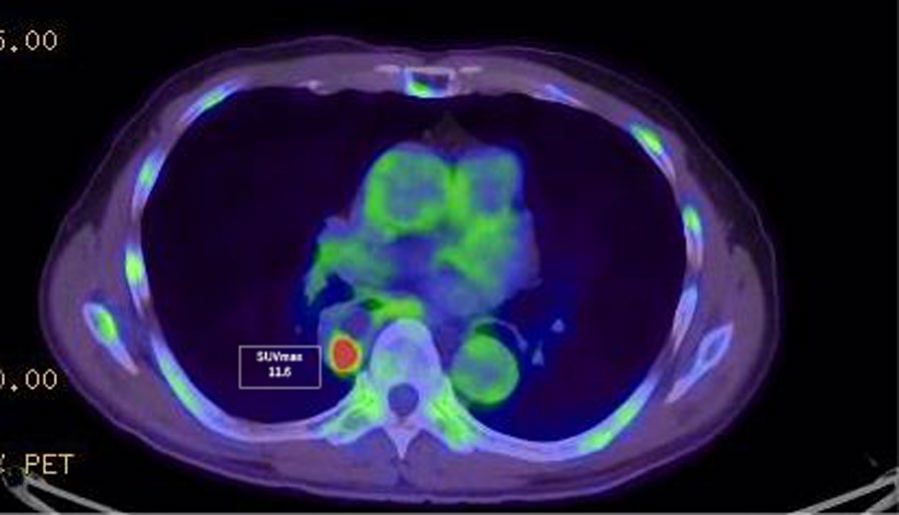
Positron emission tomography–computed tomography image showing fluorodeoxyglucose accumulation in the middle thoracic esophageal wall
